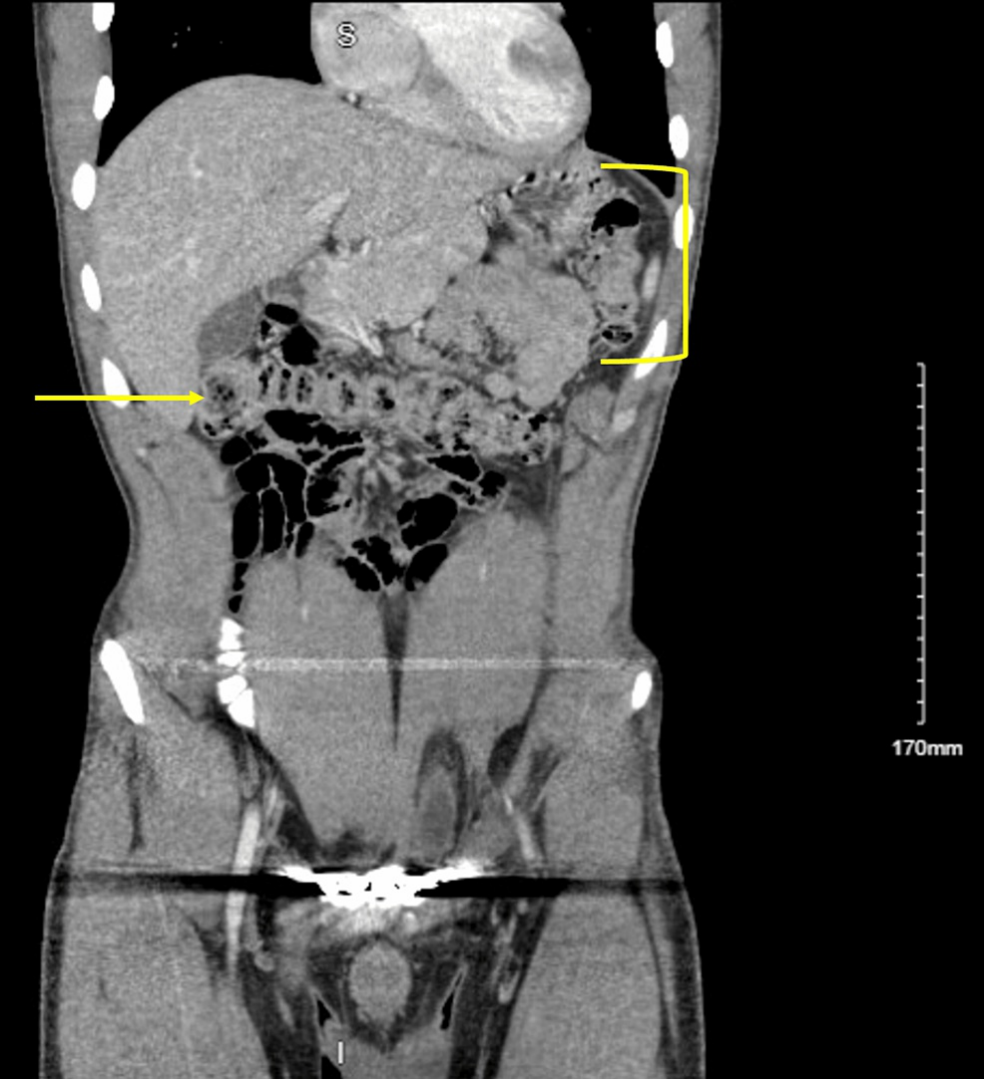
CT abdomen and pelvis demonstrating the relationship between the transverse colon (arrow) and one of the regions dense in filling defects, the ileum (bracket).
